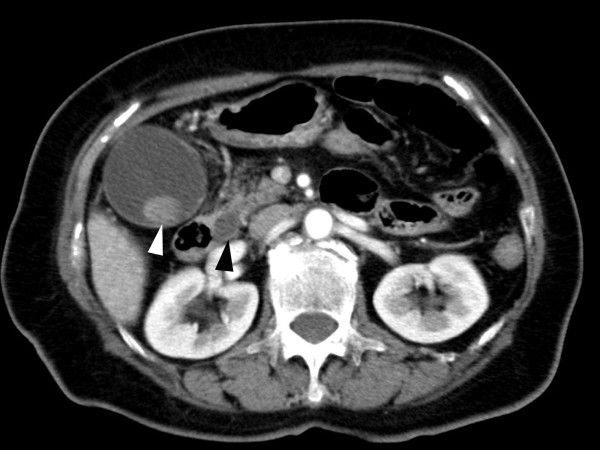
CT scan showing cholelithiasis (white arrow), choledocholithiasis and dilated common bile duct (black arrow) with a calcified stone.
